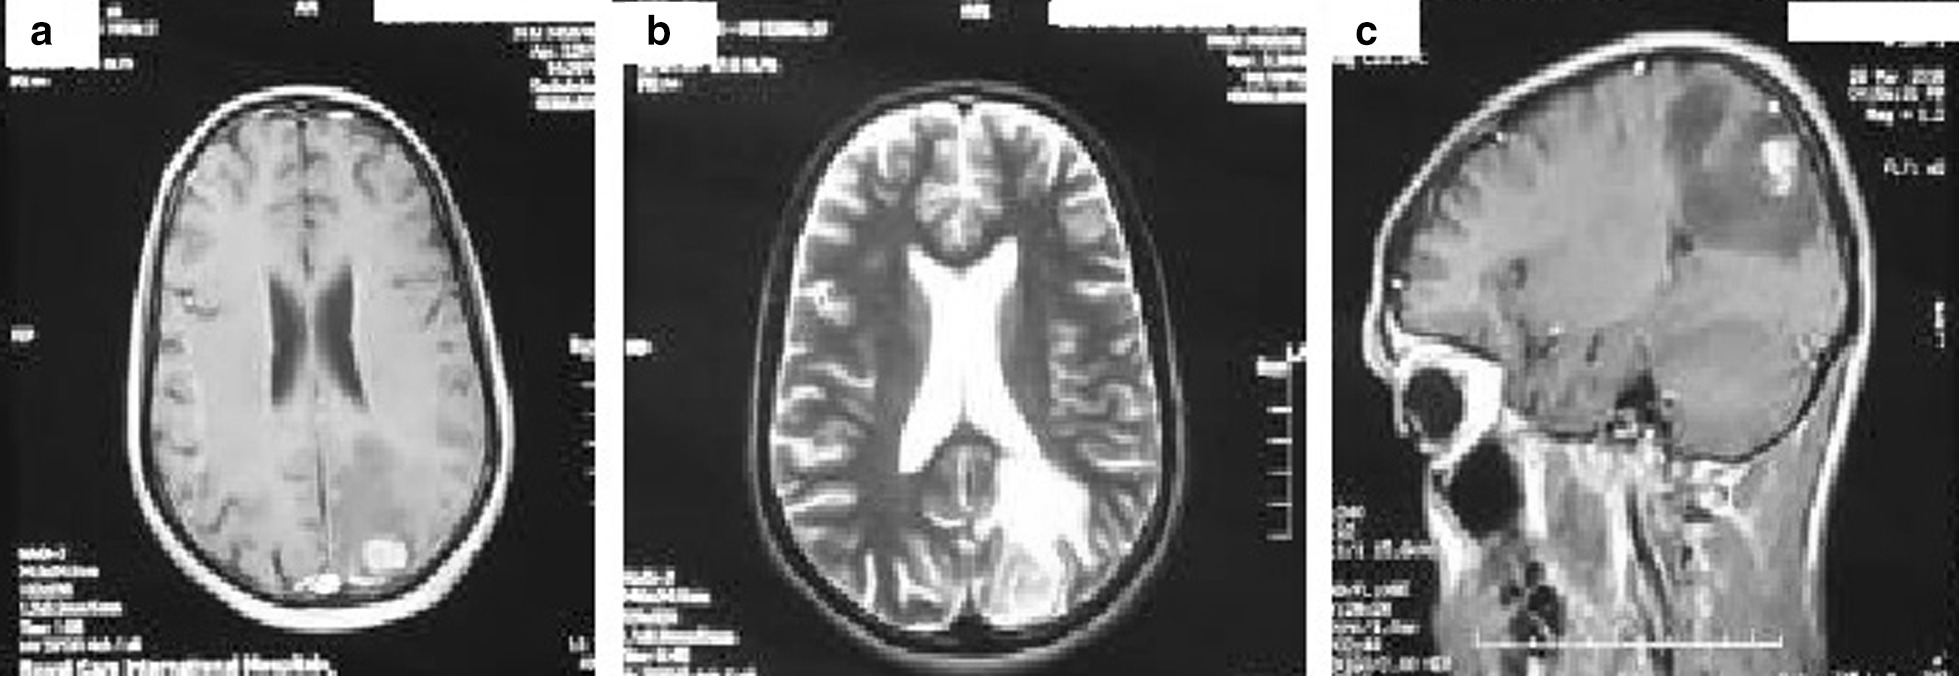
MRI brain showed left posterior parietal homogenous 1.8x1cm enhancing lesion in a) axial T1WI with peri-focal oedema. Lesion fairly defined, irregular, lobulated, intra-axial, cortical based rather than dural based, hyper-intense in b) axial T2WI and moderate size peri-lesional oedema in c) sagittal T1WI with contrast
